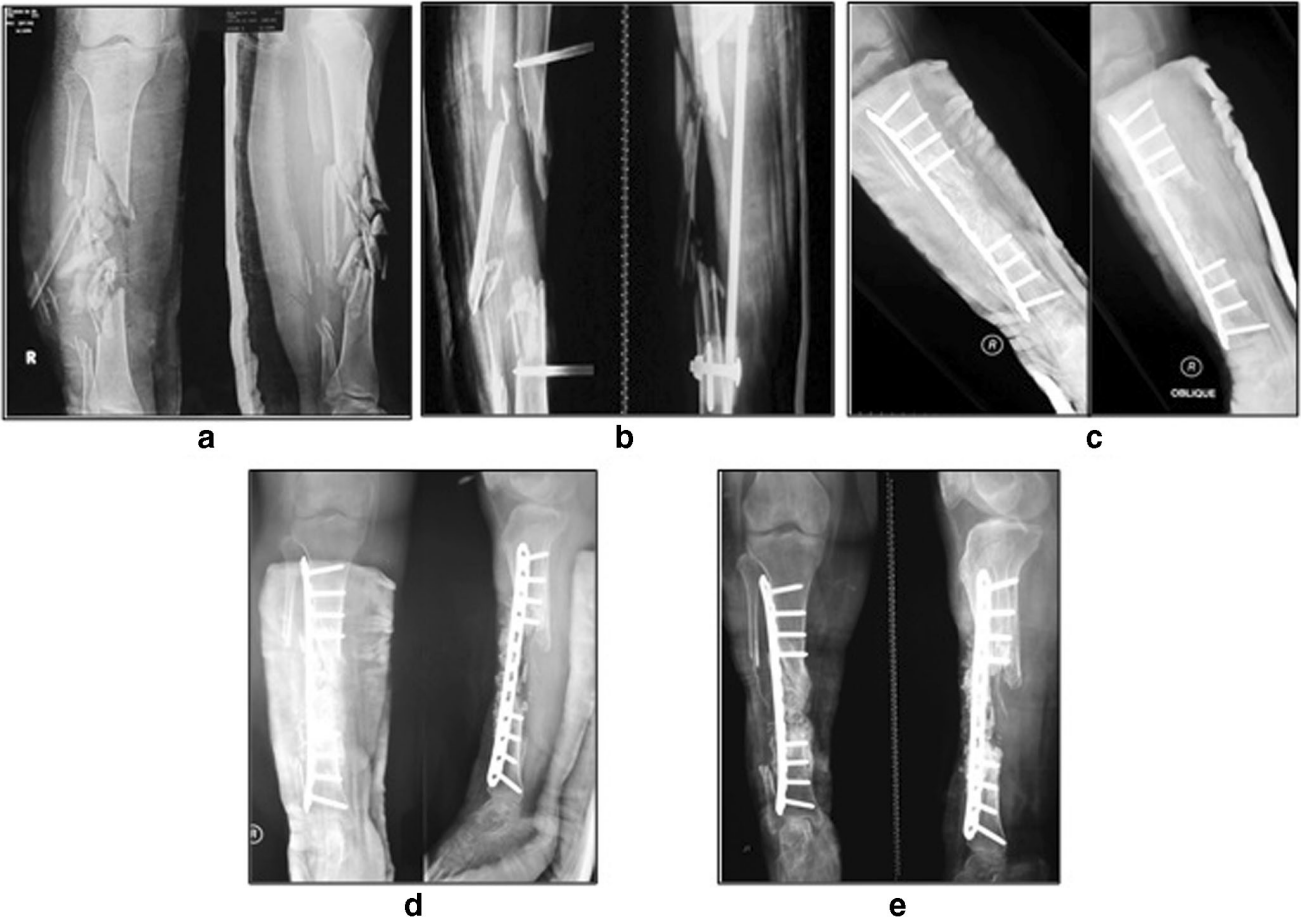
A 24-year-old female (case 3) with 12 cm BD of the tibia. a Initial radiography. b Pre-operative radiography. c Post-operative radiography. d Six-month radiography. e Fifteen-month post-operative radiography
